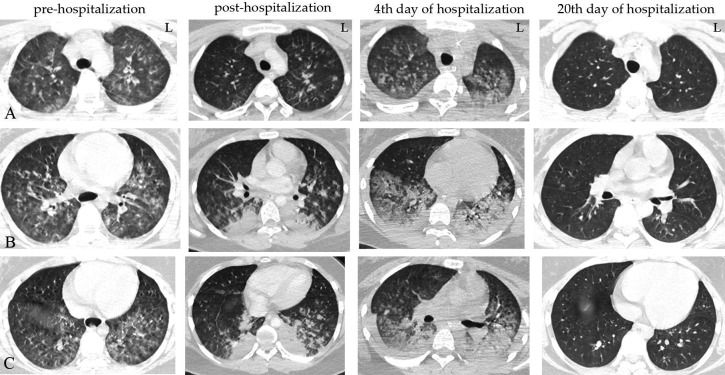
The child's prehospital lung CT showed diffuse lesions in both lungs, and the possibility of hemorrhage from lung injury was considered high; posthospital follow-up lung CT showed pulmonary hemorrhage consistent with bilateral pulmonary hemorrhage (the lower lobes of both lungs were more solid than before); on day 4, lung CT showed exudative lesions in both lungs (which were more numerous than before) and a small amount of pleural effusion on the right side; and on day 20, lung CT showed no apparent abnormality. (Note: row A is the upper lobe of the lung, row B is the middle lobe of the lung, and row C is the lower lobe of the lung).
